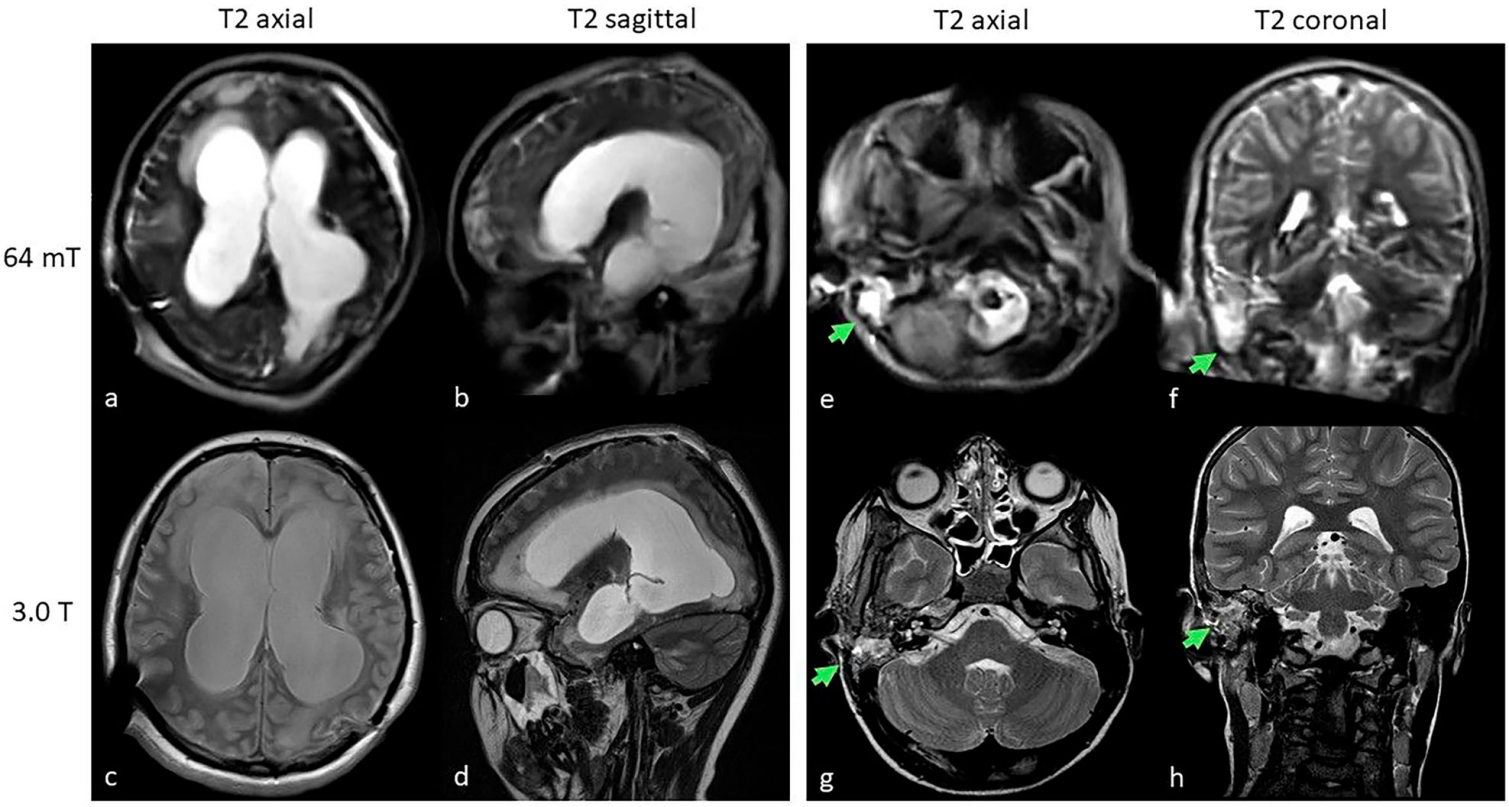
Utility of T2-weighted low-field MRI at 0.064-T (top row) acquired axial, sagittal, and coronal reconstructions in two different patients (Patient 1, a–d; Patient 2, e–h) compared to standard of care 3.0-T MRI (bottom row). Hydrocephalus was readily visualized on low-field MRI (a, b; dilated hyperintense ventricles) and confirmed on 3.0-T (c, d) in a 14-year-old female patient. Patient 2 (8-year-old male) demonstrated T2 hyperintensities of the right mastoid cells (arrows) on low-field (e, f) and confirmed on high-field MRI on the same day (g, h). While image quality on the 0.064-T Hyperfine Swoop® is not as sharp as on the high-field system, it was sufficient to provide a working diagnosis and initiate further testing in both cases
